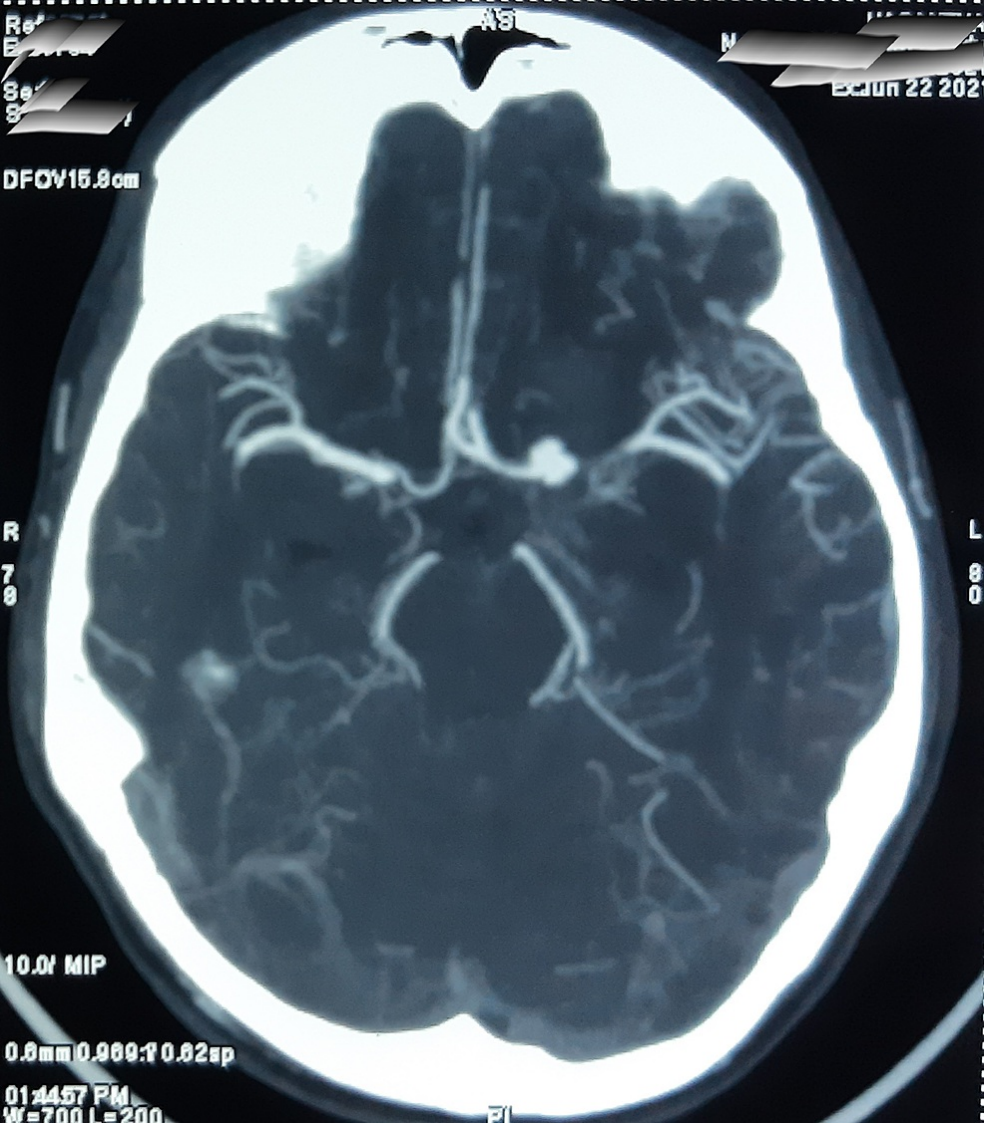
Contrast CT angiogram demonstrating left internal carotid artery aneurysm
